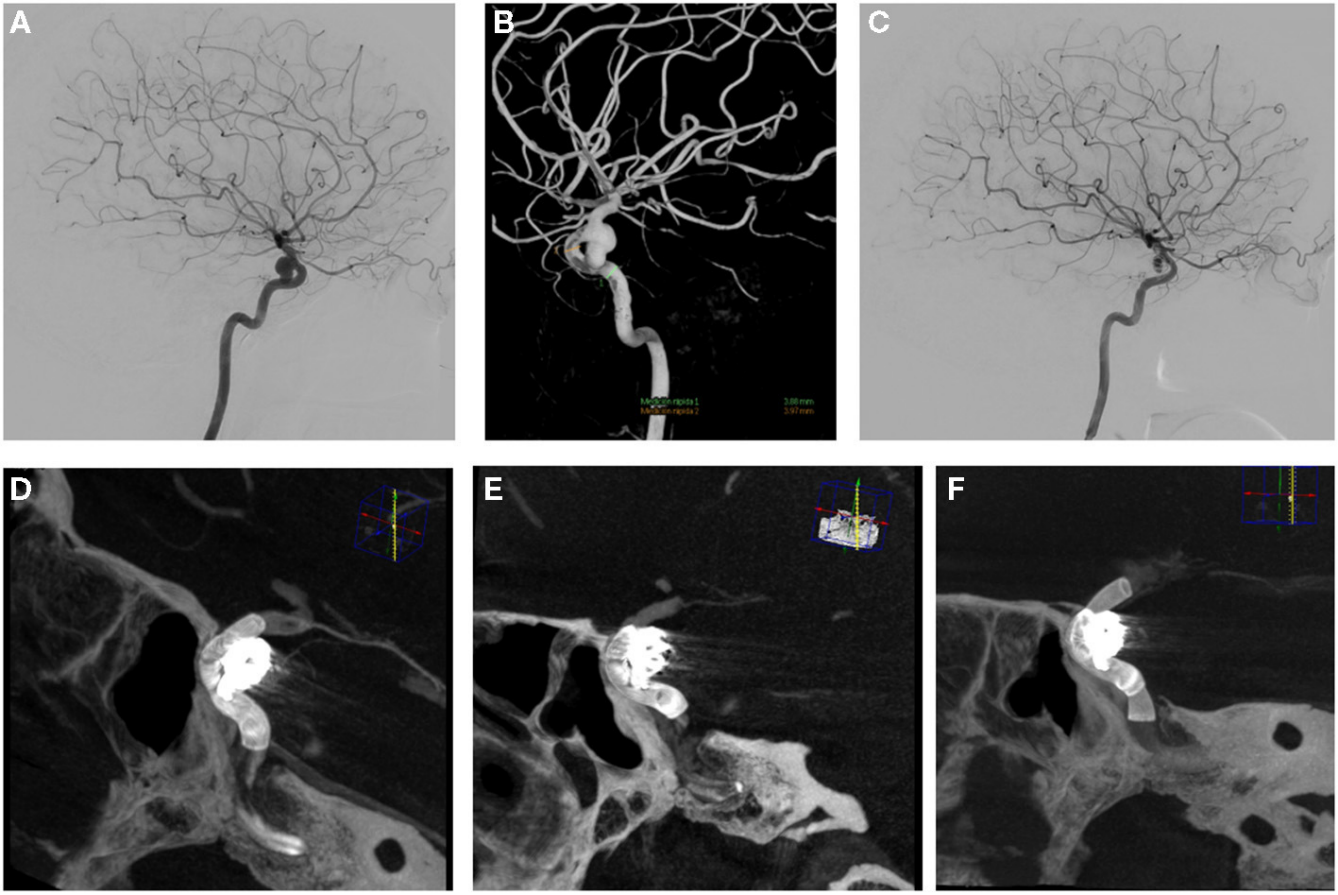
A 52-year-old woman referred for embolization of an incidental aneurysm. Digital subtraction angiography (A) and 3D rotational angiography (B) show a wide-neck left para-ophthalmic aneurysm. Stent deployment (SV 4 × 20 mm) and compartmental coiling (semi-jailing technique) was performed (C). A Vaso CT shows adequate stent apposition to the vessel covering of the aneurysm neck (D). A follow-up at 3 months, shows shortening of SV without completely covering the aneurysmatic neck (E). An overlapping 4 × 25 mm SV was deployment covering of the aneurysm neck (F).
